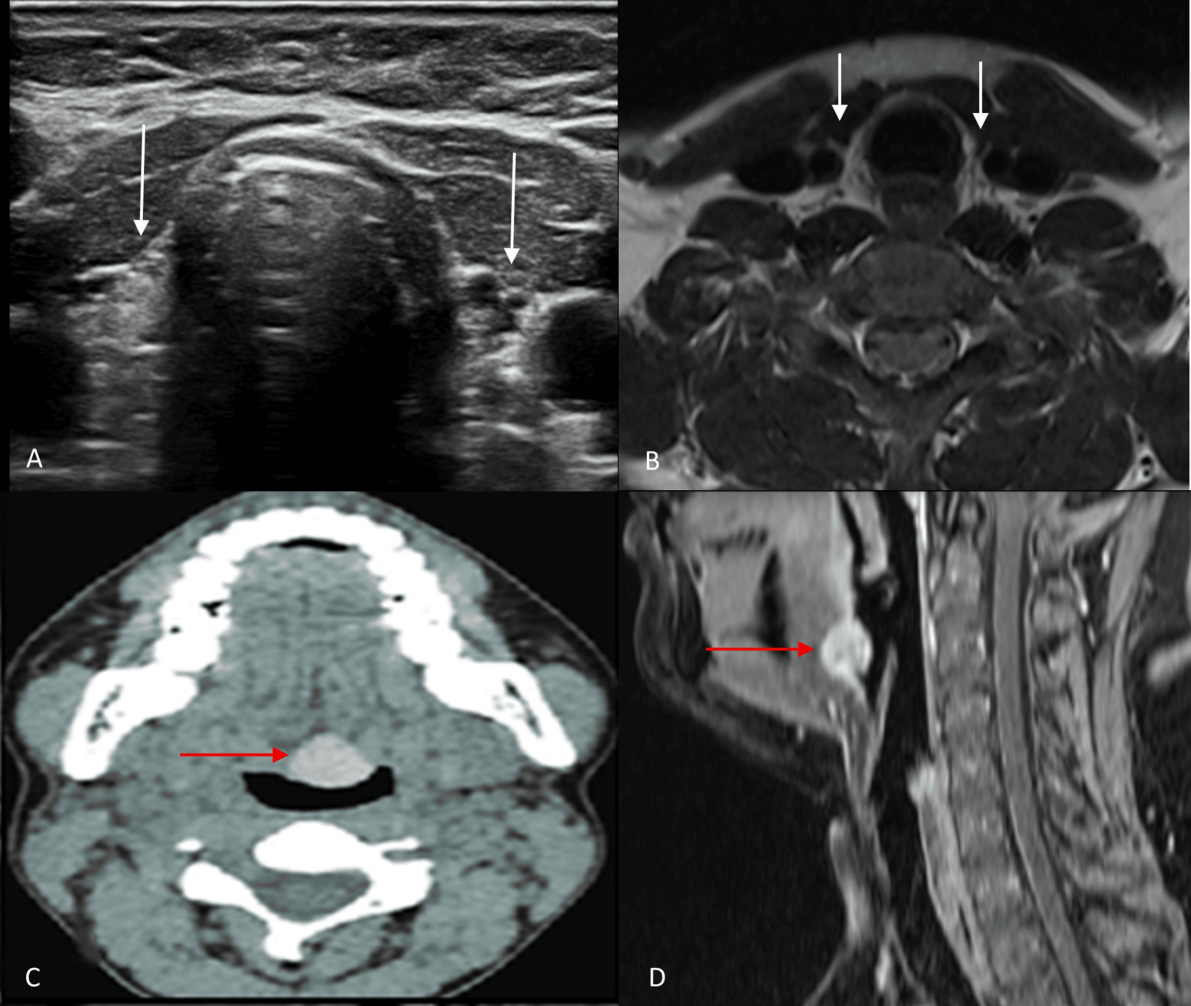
Ultrasound, CT, and MRI images of the thyroid region. (A) Gray-scale ultrasound image and (B) axial T2-weighted MRI of the thyroid region show non-visualization of the thyroid gland in its normal anatomical position (white arrows). (C) Non-contrast CT shows a hyperdense soft tissue mass at the base of the tongue (red arrow). (D) Mid-sagittal section of the post-contrast T1-weighted image shows homogenously enhancing mass at the base of the tongue (red arrow), likely representing lingual thyroid. CT, computed tomography; MRI, magnetic resonance imaging
